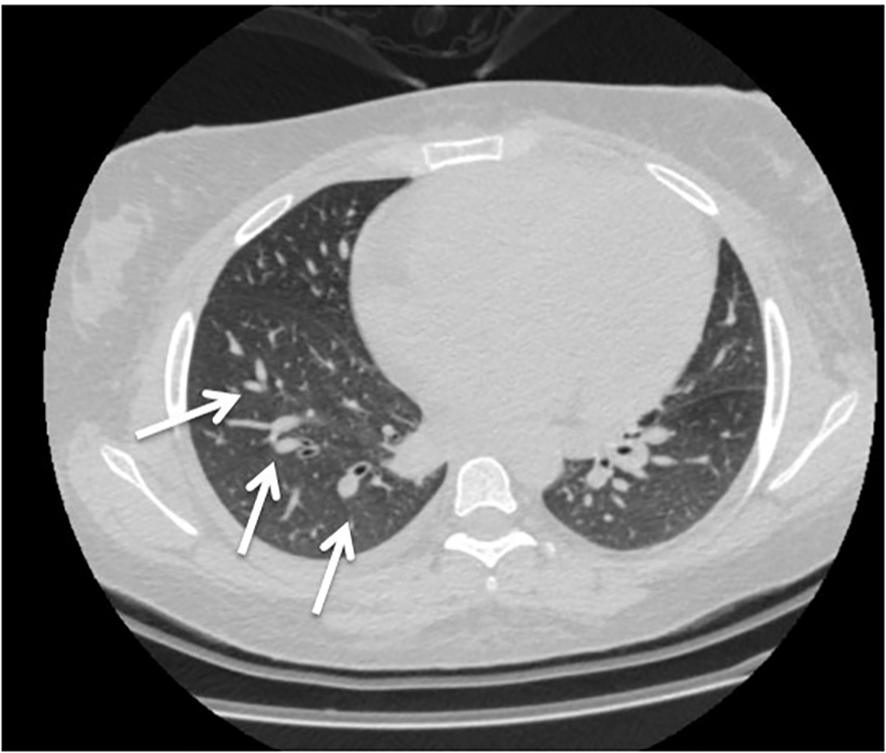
Computed tomography (CT) revealed bilateral mild pleural effusion and nodular consolidation in the lower right lobe encircled by ground-glass opacities (white arrow). She was admitted for additional assessment and treatment.
